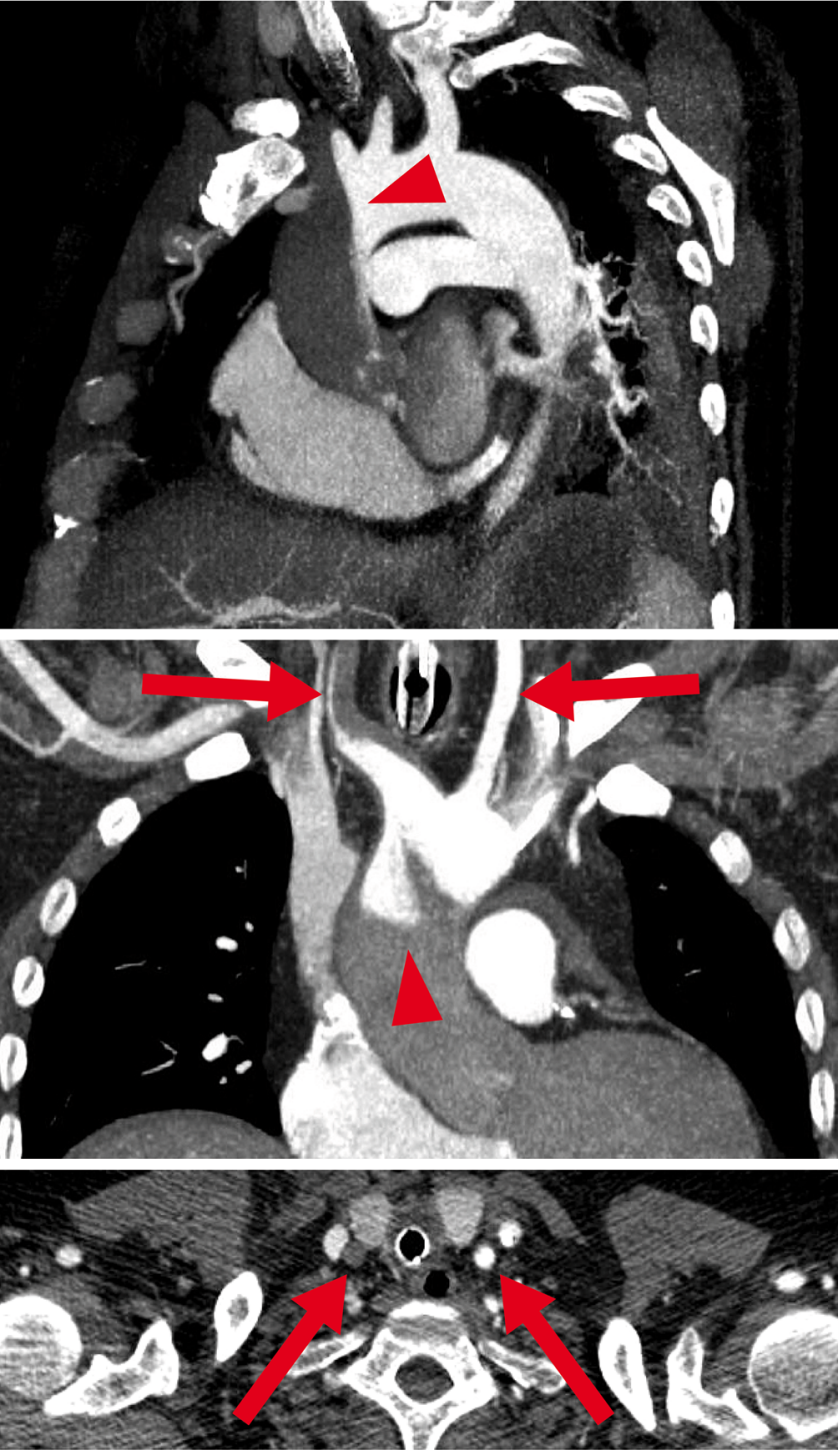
Watershed phenomenon during VA-ECMO. Computed tomography. Antegrade blood flow (*low contrast*) from the heart competes with retrograde blood flow (*high contrast*) from the ECMO in the aorta, resulting in a watershed phenomenon (*arrowhead*). Here computed tomography of a patient with pulmonary embolism and reduced cardiac output demonstrates a rather proximal watershed, leading to perfusion of the right carotid artery with “heart blood” (*dark*) and the left carotid artery with “ECMO blood” (*bright, arrows*). *Upper panel:* sagittal oblique maximum intensity projection (MIP); *middle panel:* coronal oblique MIP; *lower panel*: transverse plane. (From Napp et al. [36]; © L. C. Napp, C. Kühn, M. M. Hoeper et al. 2015. This publication is an open access publication, available on springerlink.com)
